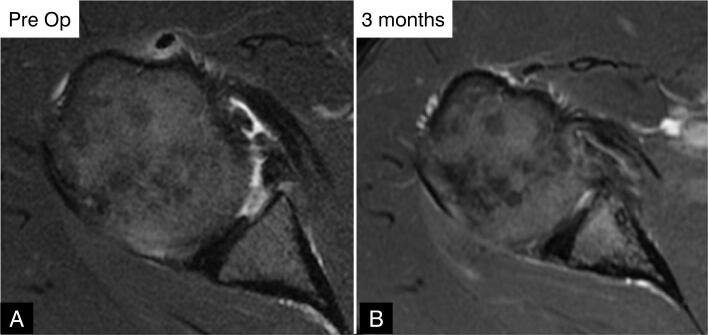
Axial MRI scan of the left shoulder. (A) Preoperative image showing a detached, incomplete labrum and (B) 3 months postoperatively showing an intact but heterogeneous labrum (grade I nodular hypersignal).
